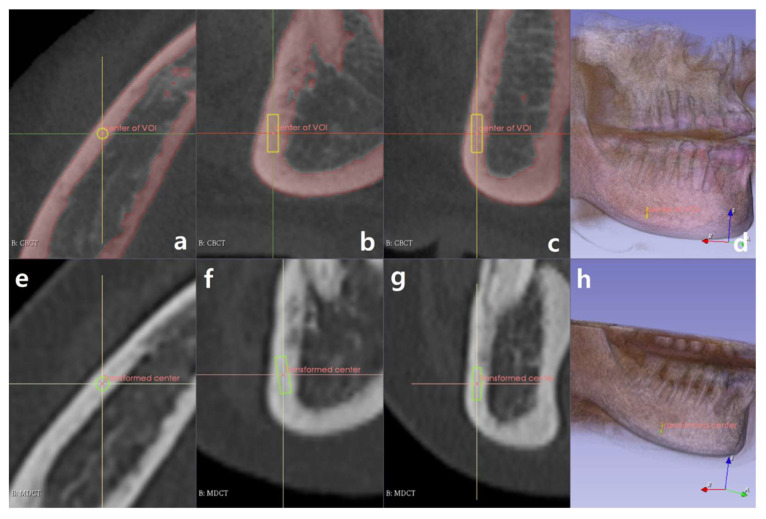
MPR views of DE CBCT and MDCT. Yellow perimeters and green perimeters indicate the selected region-of-interest (ROI) and transformed ROI, respectively: (a) axial view, (b) sagittal view, (c) coronal view, (d) 3D volume rendering of the DE CBCT image, (e) axial view, (f) sagittal view, (g) coronal view, and (h) 3D volume rendering of the MDCT image.
